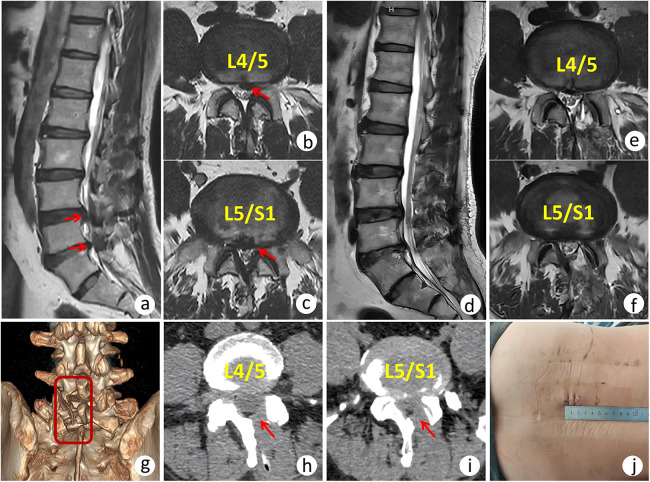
A 54-year-old male patient with L4/5 and L5/S1 AT-LDH treated by AUSS. (a–c) Preoperative MRI demonstrating disc herniation at L4/5 and L5/S1 levels (arrow). (d–f) Postoperative MRI demonstrating complete removal of the herniated disc. (g) Postoperative 3D reconstruction demonstrating partial laminectomy at L4 and L5 levels (boxed area). (h–i) Axial CT scan demonstrating laminar fenestration (arrow). (j) Postoperative incision length.
